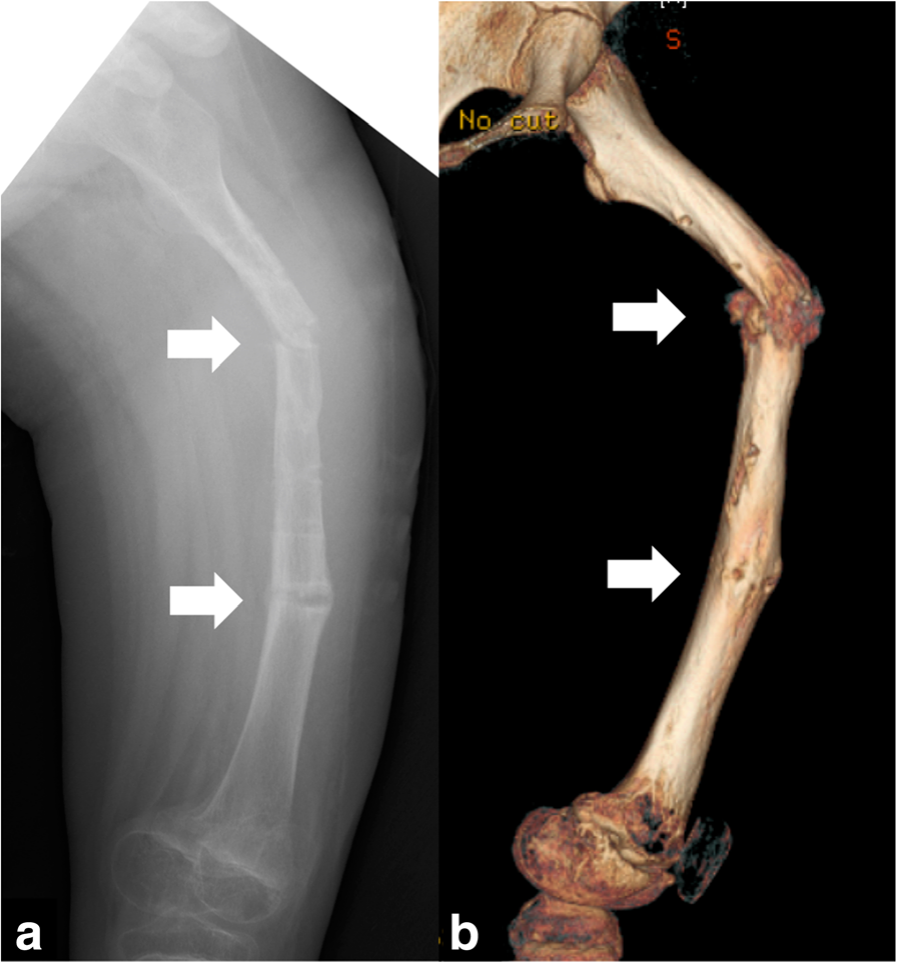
a A radiograph of the left femur presenting the two fractures at half-pin insertion sites. (arrows) (b) The three- dimensional reconstruction of the left femur presenting the two fractures at half-pin insertion sites. (arrows)
